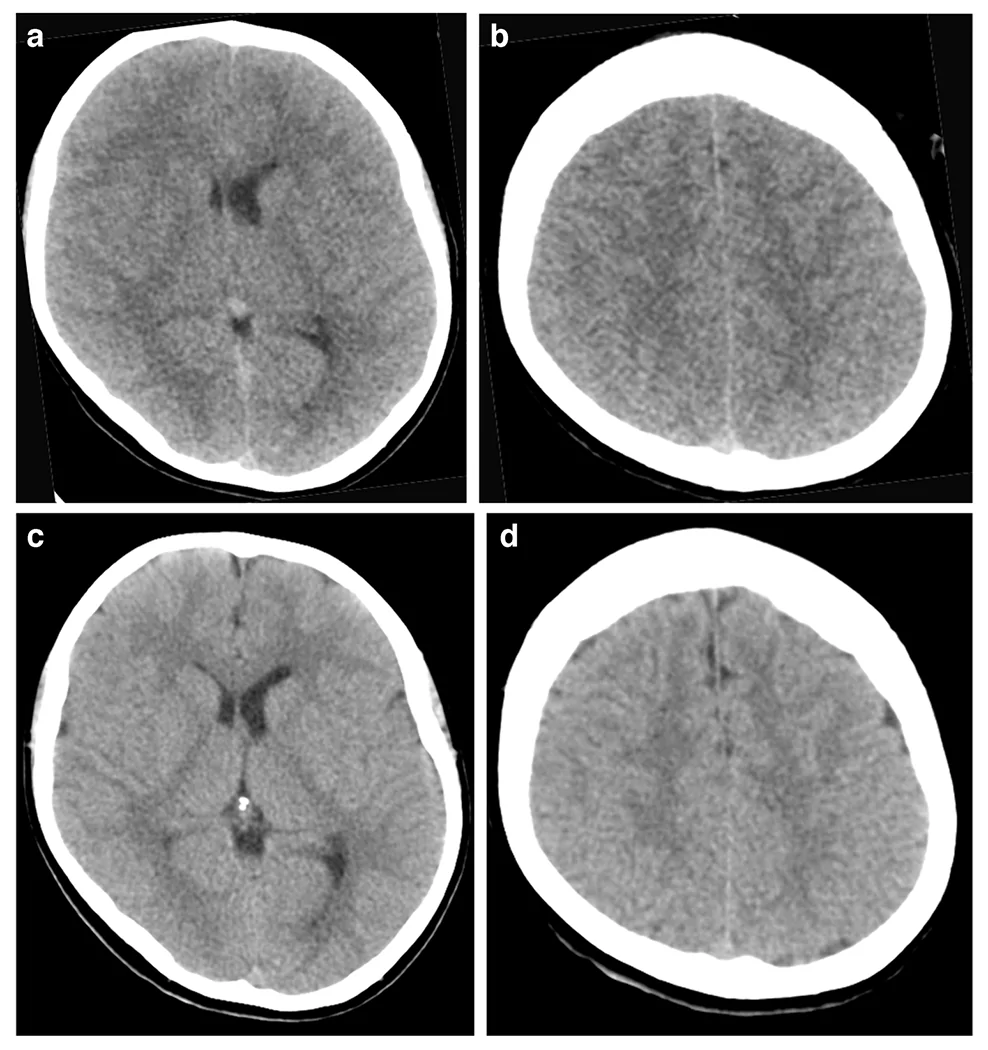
Fourteen-year-old female with diabetic ketoacidosis and cerebral edema. a, b Non-contrast head computed tomography images at the level of the (a) lateral ventricles and (b) vertex show partially effaced slit-like right lateral ventricle and widespread effacement of the cerebral sulci. c, d Following treatment with mannitol and hypertonic saline, repeat non-contrast head computed tomography images at the level of the (c) lateral ventricles and (d) vertex show normalization of the cerebrospinal fluid spaces, confirming decreased or resolved cerebral edema
